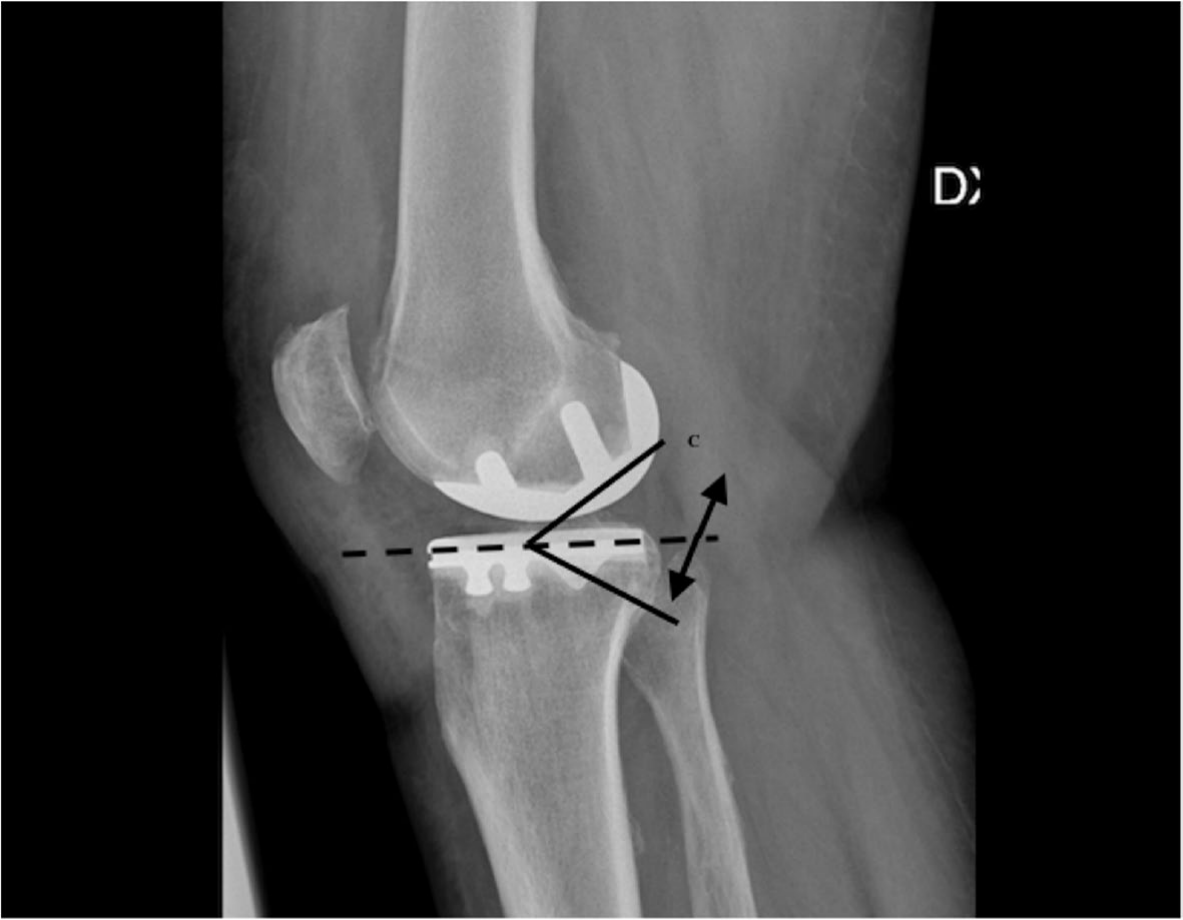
Lateral radiograph, right knee; Radiological parameters measured: anteroposterior tibial slope (C)
